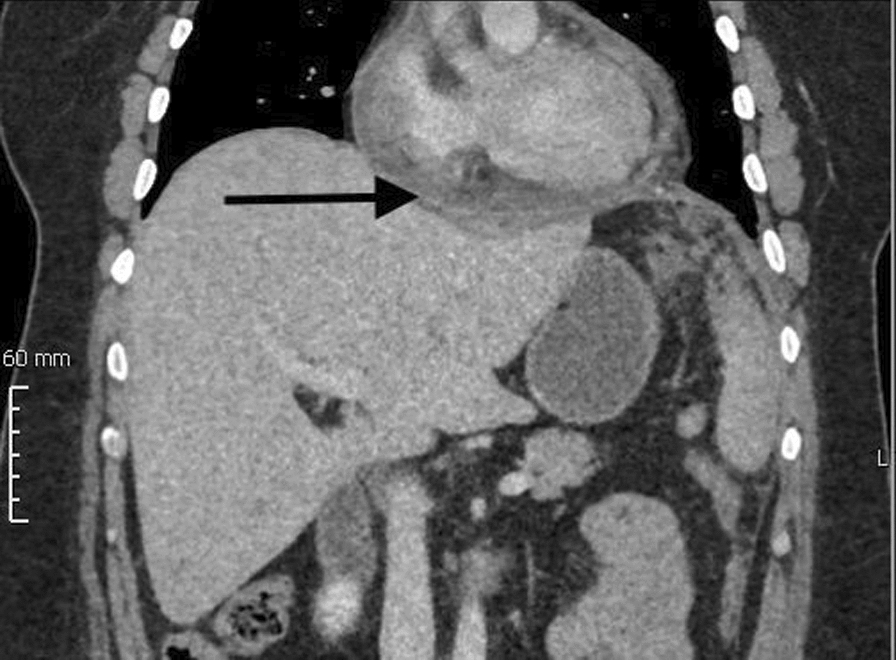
Computed tomography coronal plane images, black arrow points to transdiaphragmatic rupture of a large hepatic abscess into the pericardial space
